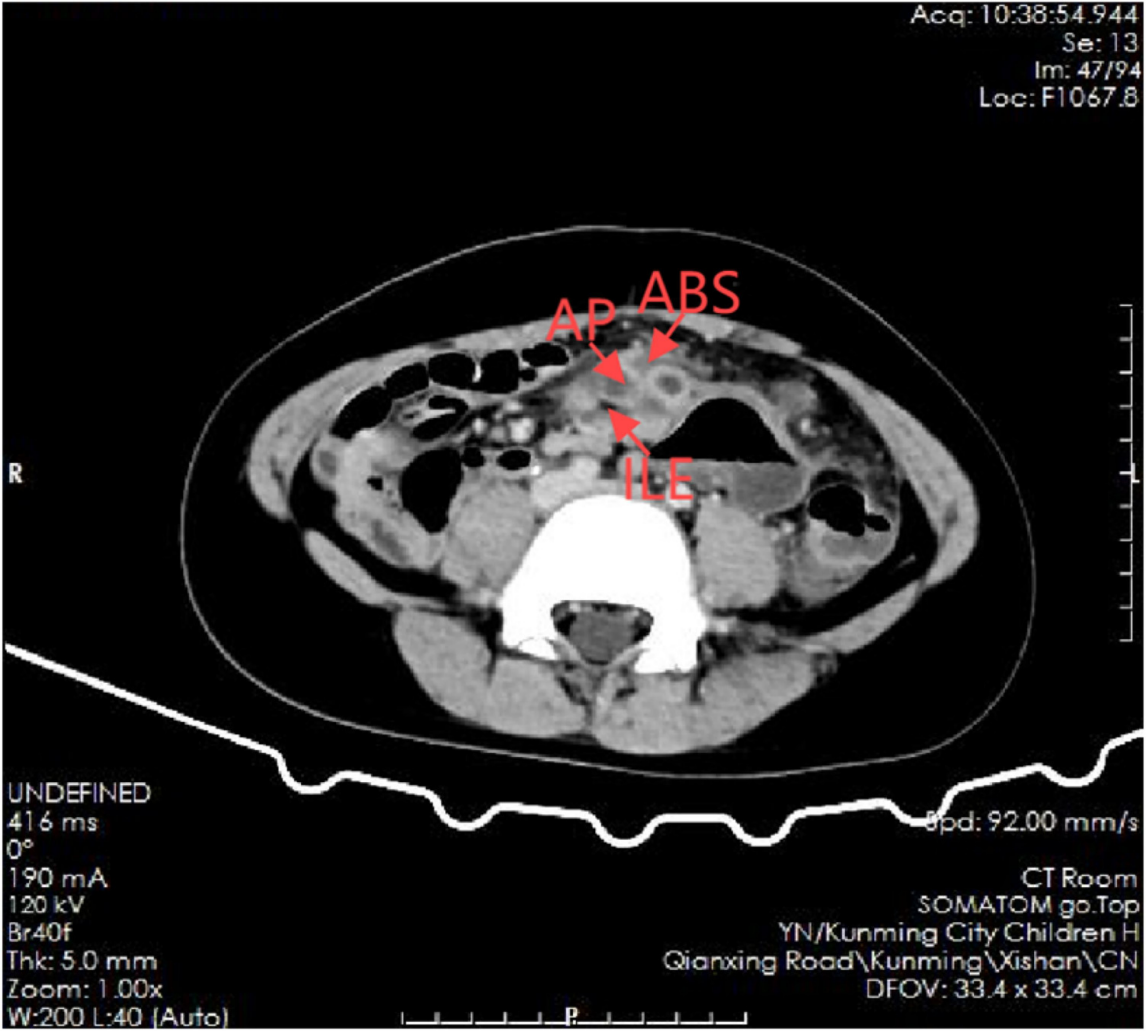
Contrast-enhanced CT showing a left-sided ileocecal region (arrow) and inflammatory changes consistent with left-sided appendicitis. AP, appendix; ABS, abscess; ILE, ileocecal region.
